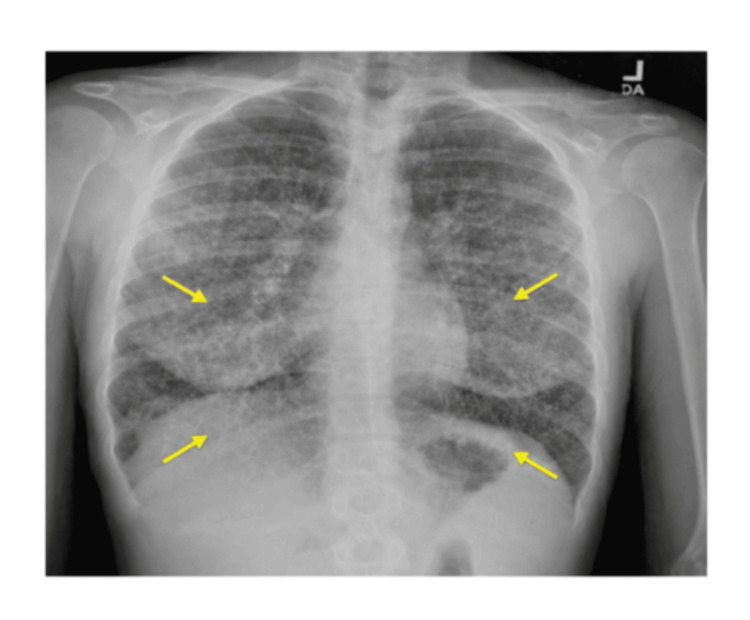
Initial chest X-ray showing bilateral micronodular opacities Frontal chest radiograph demonstrates diffuse, bilateral micronodular opacities (arrows) involving the mid to lower lung zones, more pronounced in the perihilar and peripheral regions. Cardiomediastinal silhouette and costophrenic angles are within normal limits.
